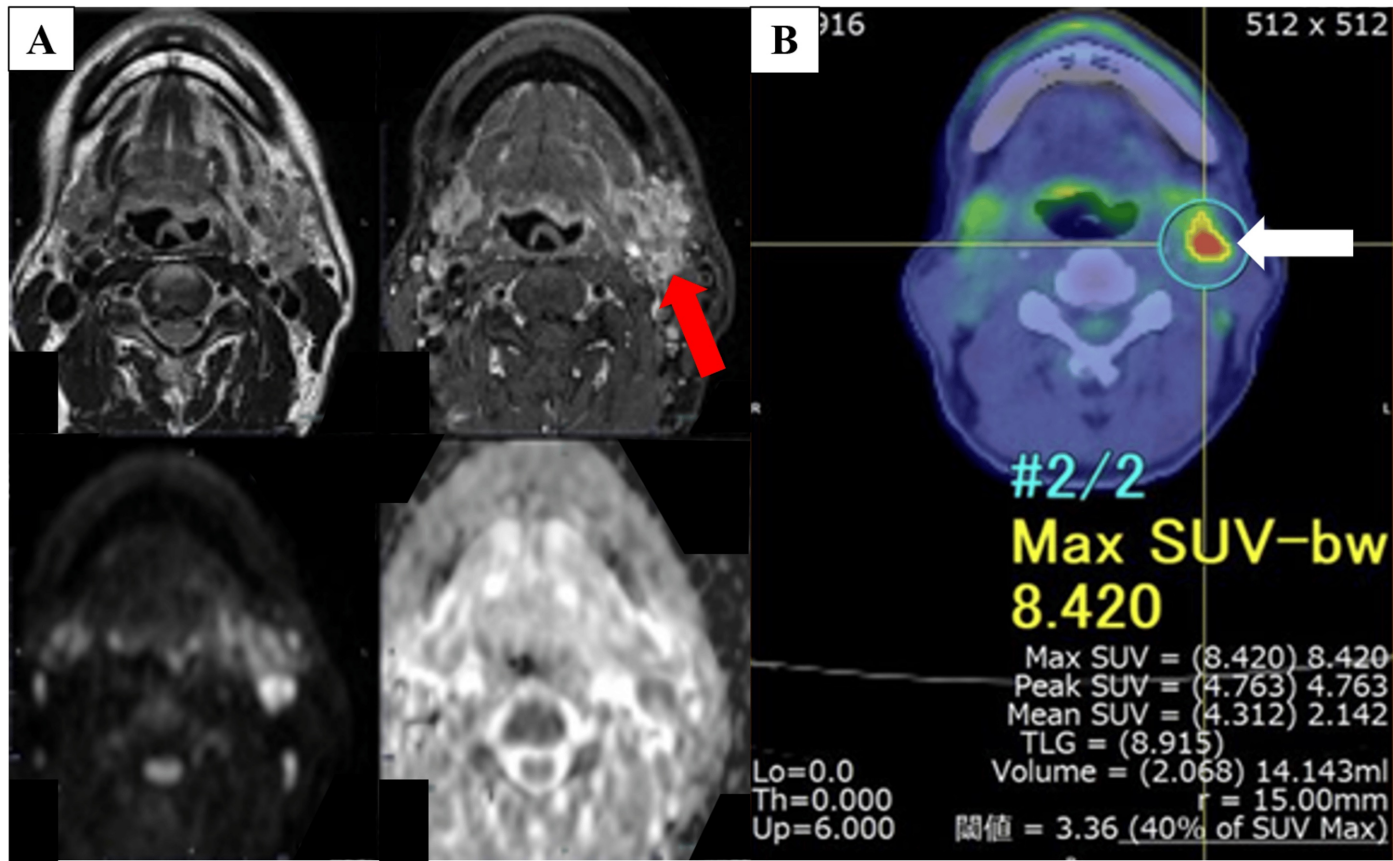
Radiologic evaluation of left tongue cancer (A) T2-weighted MRI demonstrating a hyperintense lesion (bright on T2; red arrow) at the left tongue margin with contrast enhancement and restricted diffusion, extending toward the lingual septum. (B) PET-CT revealing FDG uptake (increased glucose-tracer uptake indicating high metabolic activity) at the primary tumor and the ipsilateral cervical lymph node (white arrow). R/L orientation and scale bars are included where applicable. The imaging supports a metabolically active primary tongue malignancy with nodal involvement. MRI: magnetic resonance imaging; PET-CT: positron emission tomography-computed tomography; FDG: fluorodeoxyglucose
